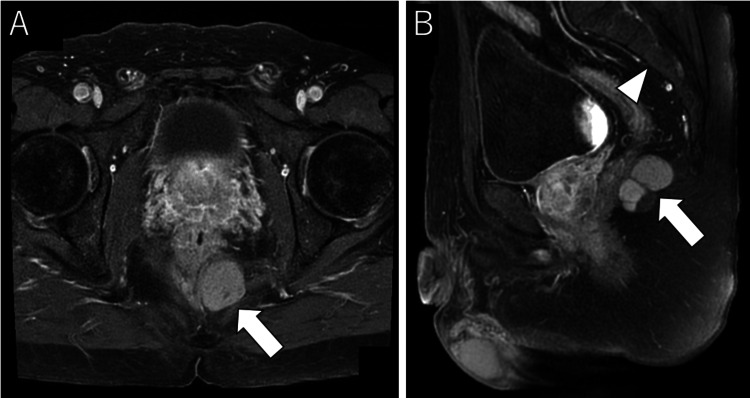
T1-weighted images of abdominal MRI (A) Axial magnetic resonance imaging (MRI) showed a high signal inside the tumor, which had an unclear contrast effect (arrow). (B) Coronal MRI revealed that the tumor (arrow) was caudal to the third sacrum (arrowhead).
